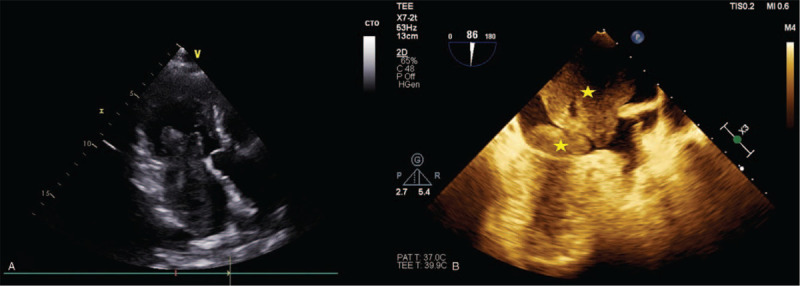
Compared with Routine TTE (A), TEE (B) could display the exact number of tumors (2, yellow stars) in the same section(Apical three-chamber heart section). The masses had no clear boundary with the endocardium or pericardium with low mobility.
